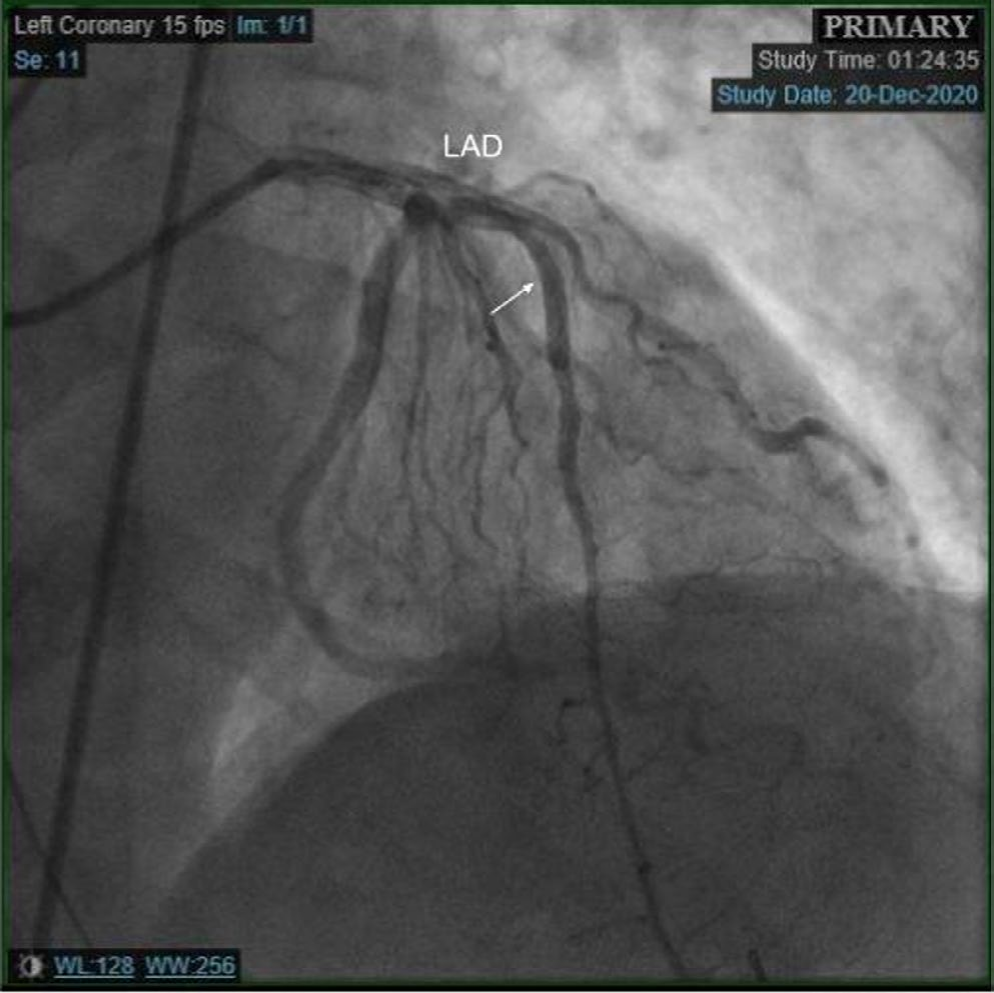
Intravascular ultrasound of the left anterior descending coronary artery without signs of atherosclerotic significant disease or lesions
